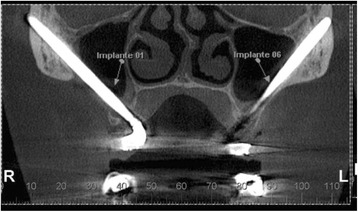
Coronal slice from the CBCT showing small exteriorization of a zygomatic implant apex
